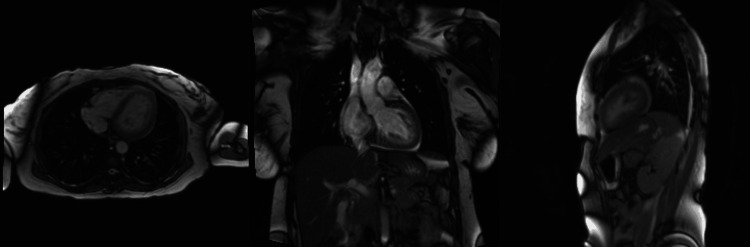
Cardiac magnetic resonance imaging (CMRI) The CMRI shows normal biventricular size and function, with mild septal T1 elevation suggesting subtle inflammation. There is minimal pericardial enhancement consistent with mild chronic pericarditis, and minimal bilateral pleural effusion, with no evidence of cardiomyopathy.
